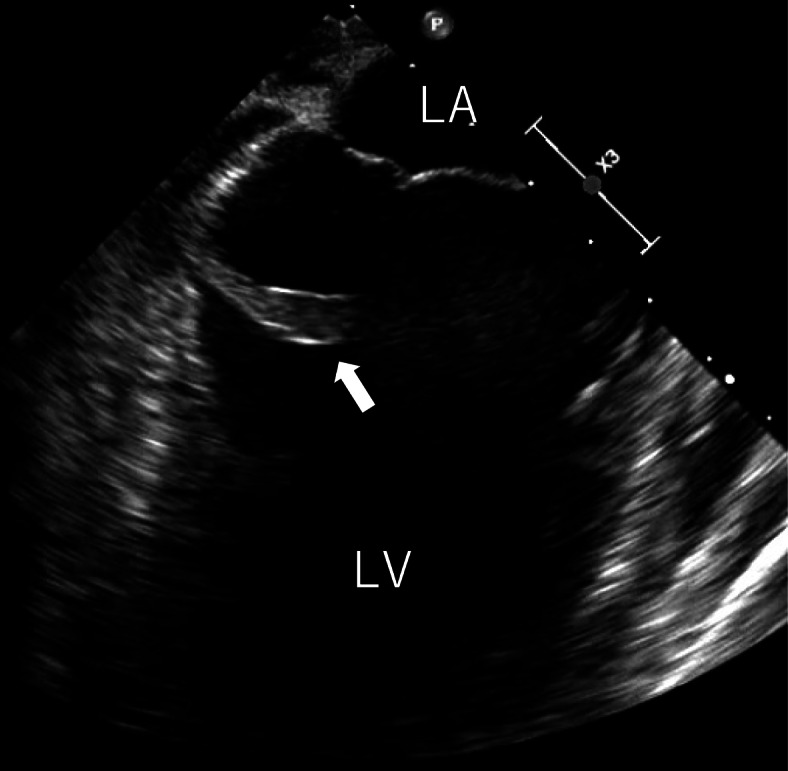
Preoperative transesophageal echocardiography findings. Transesophageal echocardiography revealed a mobile club-shaped mass of 22.0 × 8.0 mm on the basal posterior wall of the left ventricle. LA, left atrium; LV, left ventricle
